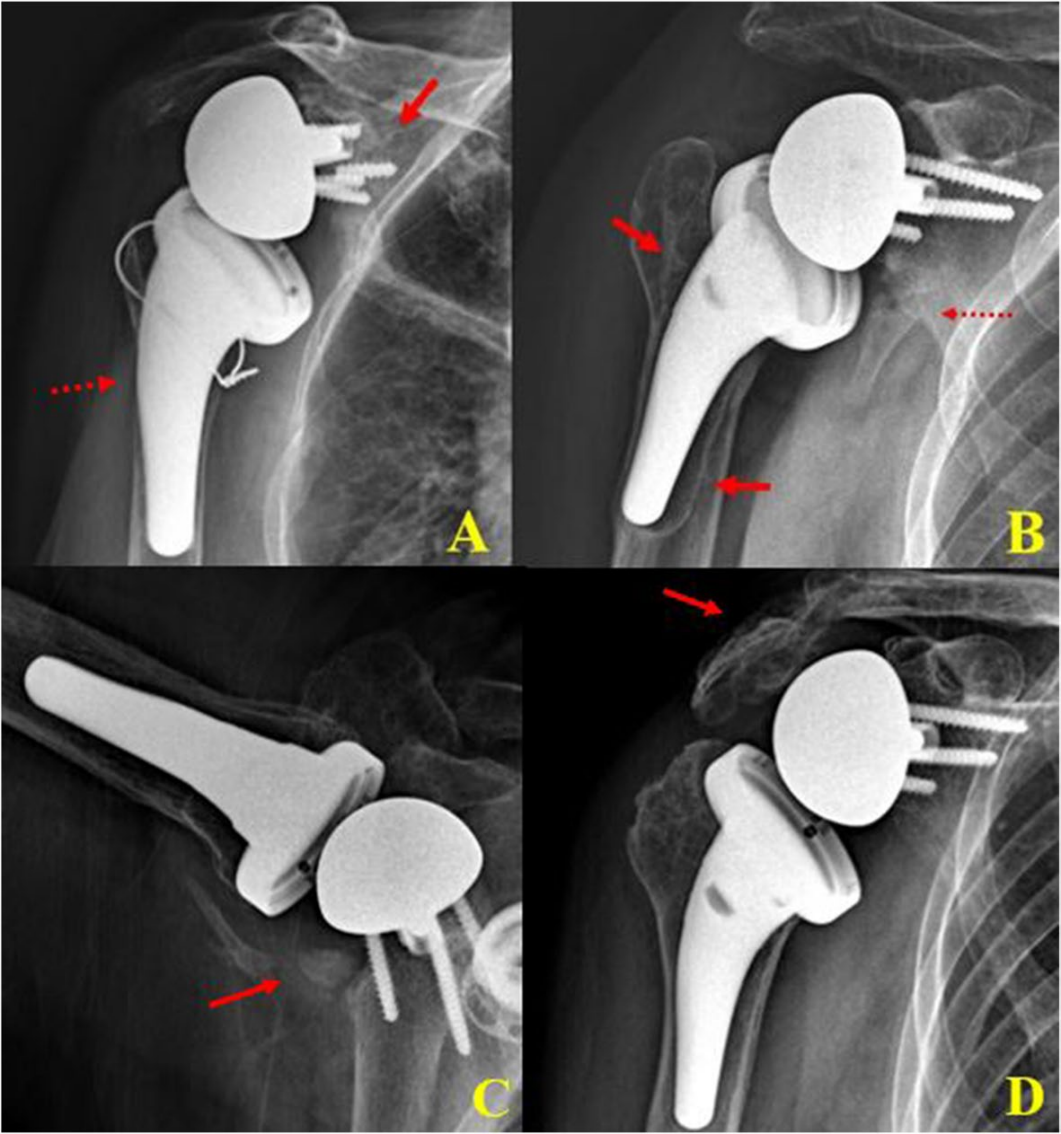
Radiologic complications of humeral lateralization reverse total shoulder arthroplasty. Glenoid loosening with broken screw (arrow) and high adaptation of the humeral stem (dotted arrow) (A). Scapular neck fracture (dotted arrow) and trauma-related humeral stem loosening (arrow) (B). Axillary lateral view showing Grade 4 scapular notching (C). Humeral stem loosening was developed in a 5-year follow-up X-ray (C). Late acromion fracture was developed and conservative treatment was done (D)
